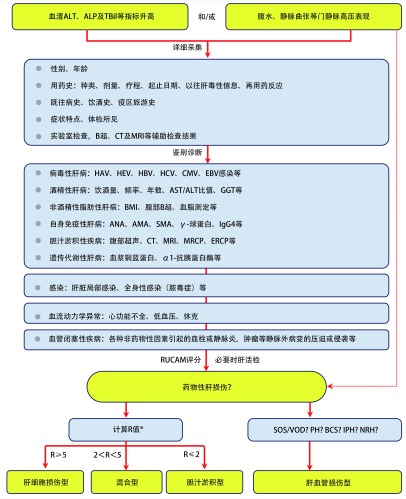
DILI诊断流程图。甲型肝炎病毒（hepatitis avirus, HAV）；戊型肝炎病毒（hepatitis E virus, HEV）；巨细胞病毒（cytomegalovirus, CMV）；EB病毒（Epstein-Barr virus, EBV）；谷氨酰基转移酶（glutamyltransferase, GGT）；身体质量指数（Body Mass Index, BMI）；B型超声（type-B ultrasonic ultrasonic test, B超）；抗核抗体（antinuclear antibody, ANA）；抗线粒体抗体（anti-mitochondrial antibody, AMA）；抗平滑肌抗体（anti-smooth muscle antibody, SMA）；磁共振胰胆管造影（magnetic resonance cholangiopancreatography, MRCP）；内镜下逆行胰胆管造影（endoscopic retrograde cholangiopancreatography, ERCP）；巴德-基亚里综合征（Budd-Chiari syndrome, BCS）；特发性门静脉高压症（idiopathic portal hypertension, IPH）；结节性再生性增生（nodular regenerative hyperplasia of the liver, NRH）；紫癜性肝病（peliosis hepatitis, PH）；肝窦阻塞综合征/肝小静脉闭塞病（sinusoidal obstruction syndrome/veno-occlusivedisease, SOS/VOD）：^*^R＝[ALT实测值/上限（upper limit of normal value, ULN）]/（ALP实测值/ULN）。 Diagnostic algorithm of DILI
